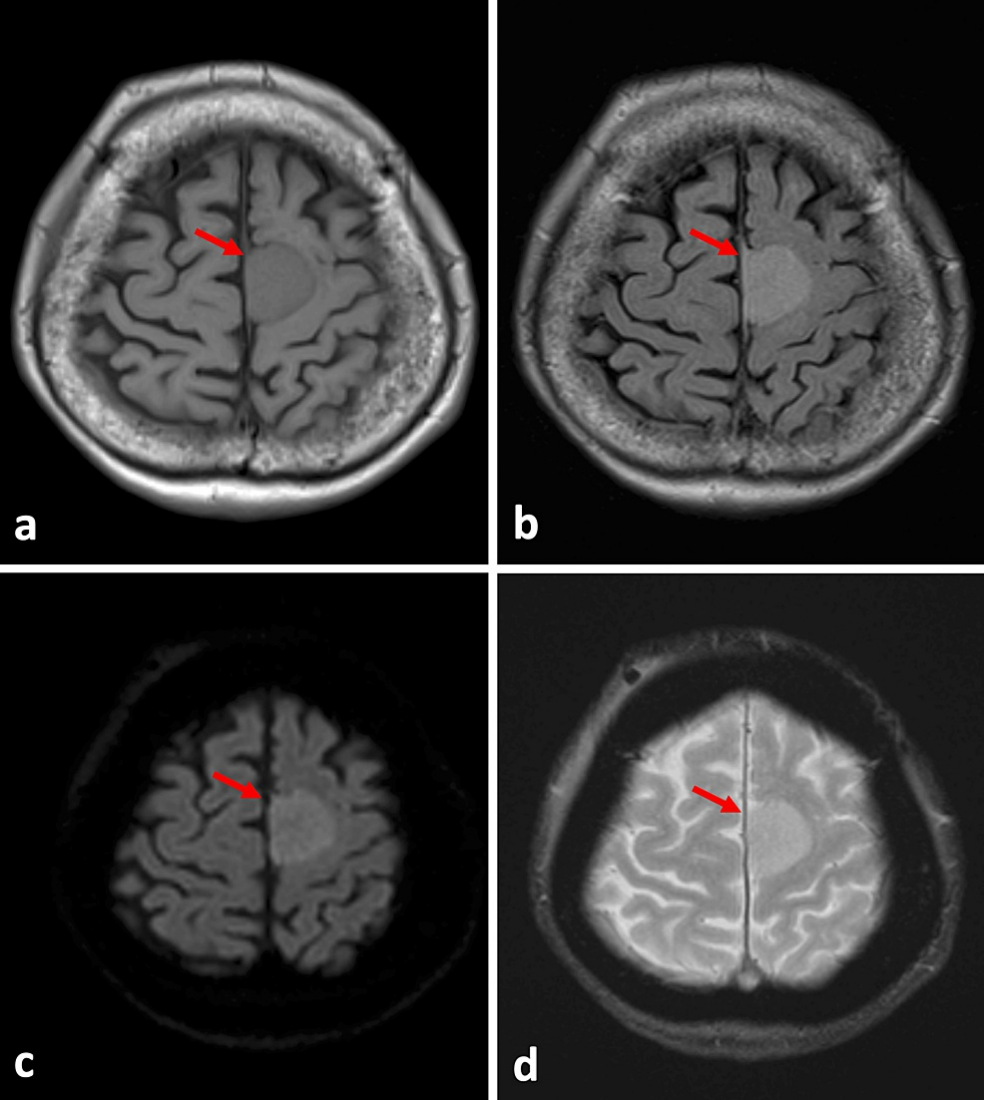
Results of magnetic resonance imaging one year before admission a: T1 weighted image; b: FLAIR; c: diffusion-weighted image; d: T2-star weighted image The tumor is in contact with the left side of the cerebral falx (red arrows). The internal signal appears uniform, and there are no indications of hemorrhage. FLAIR: fluid-attenuated inversion recovery
